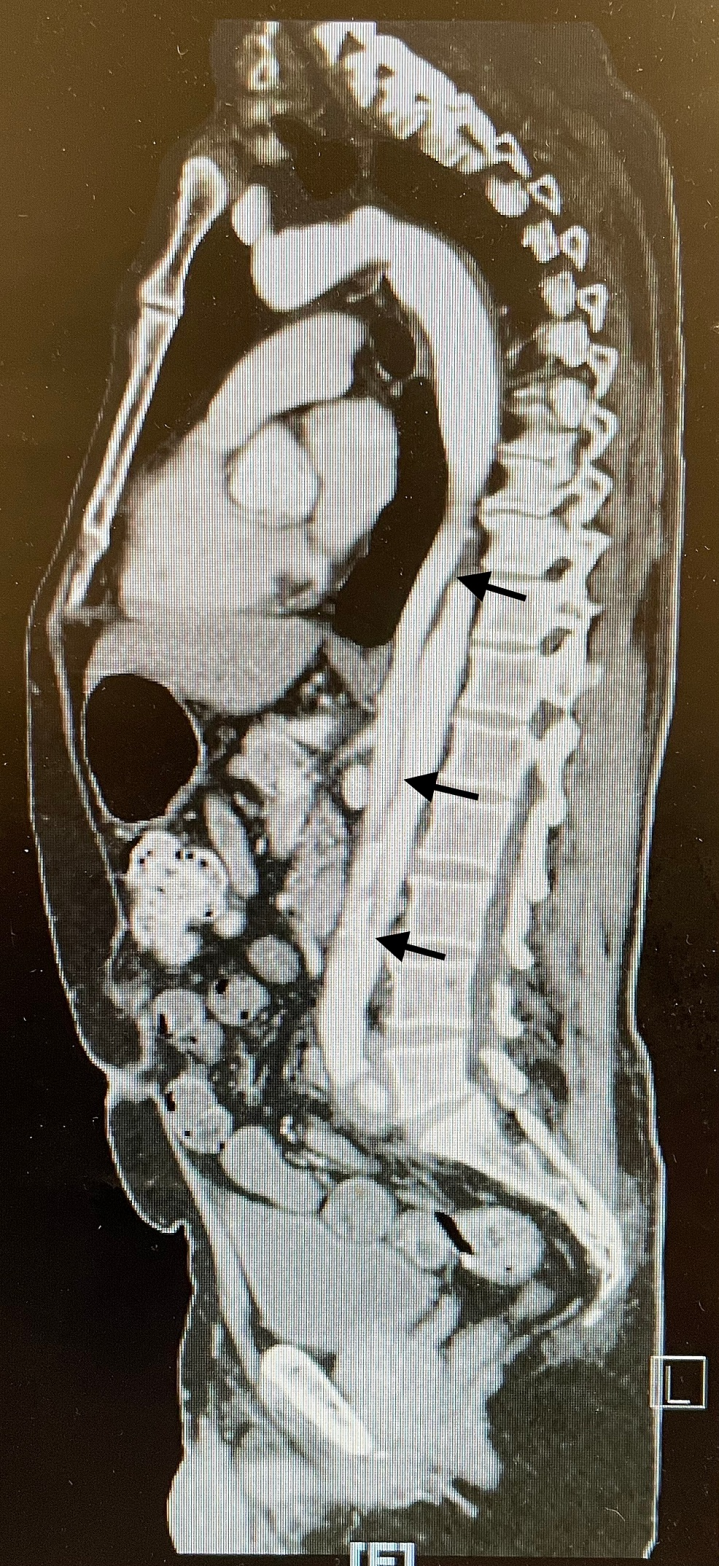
Aortic dissection as seen on CT angiogram CT: computed tomography
